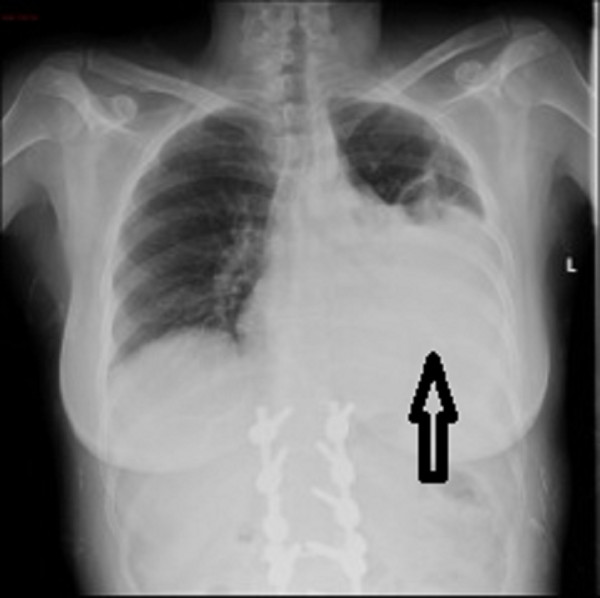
Chest x-ray. Black arrow indicates left pleural effusion.
